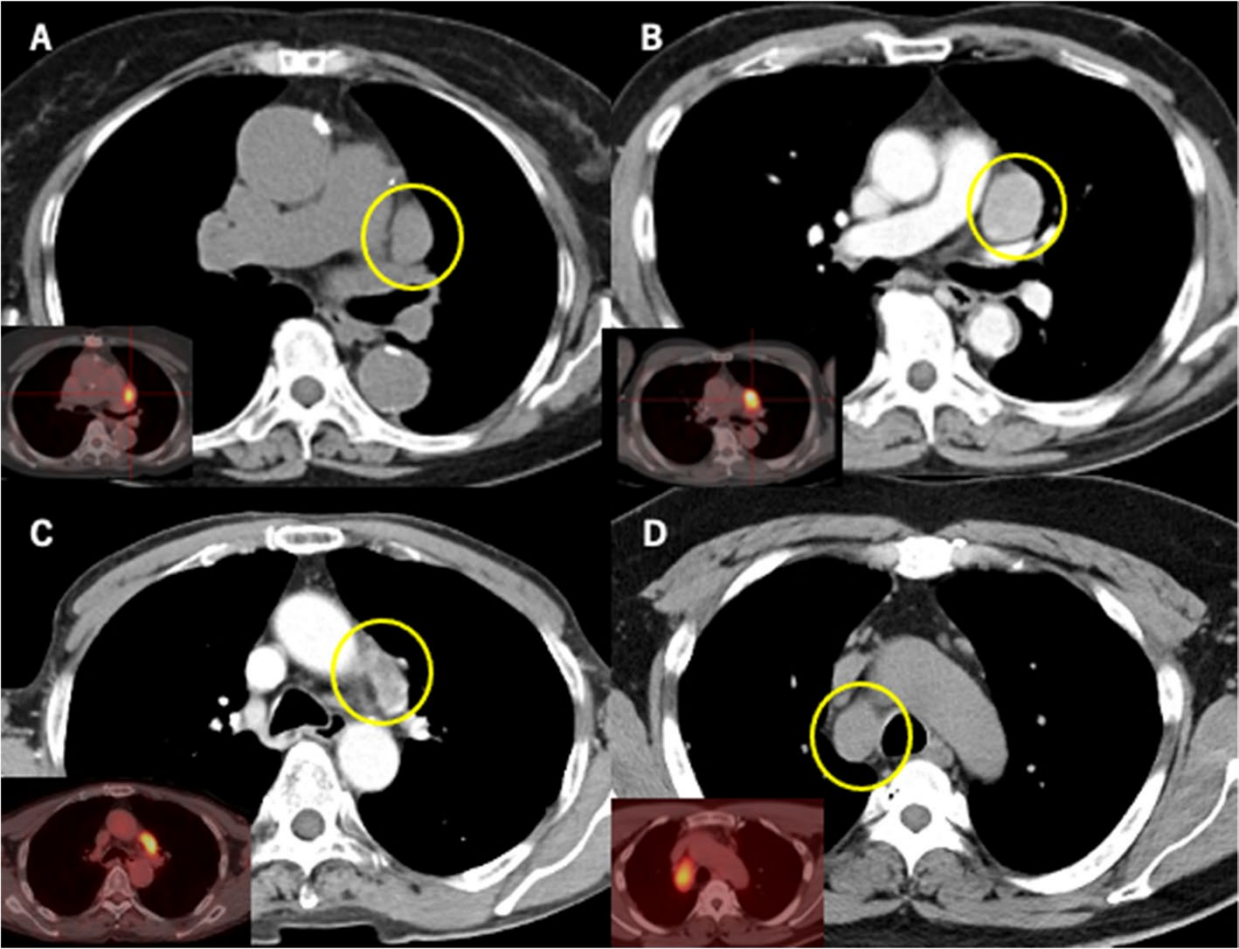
Preoperative CT (yellow circles) and PET images of Patient A (A), Patient B (B), Patient C (C), and Patient D (D)
